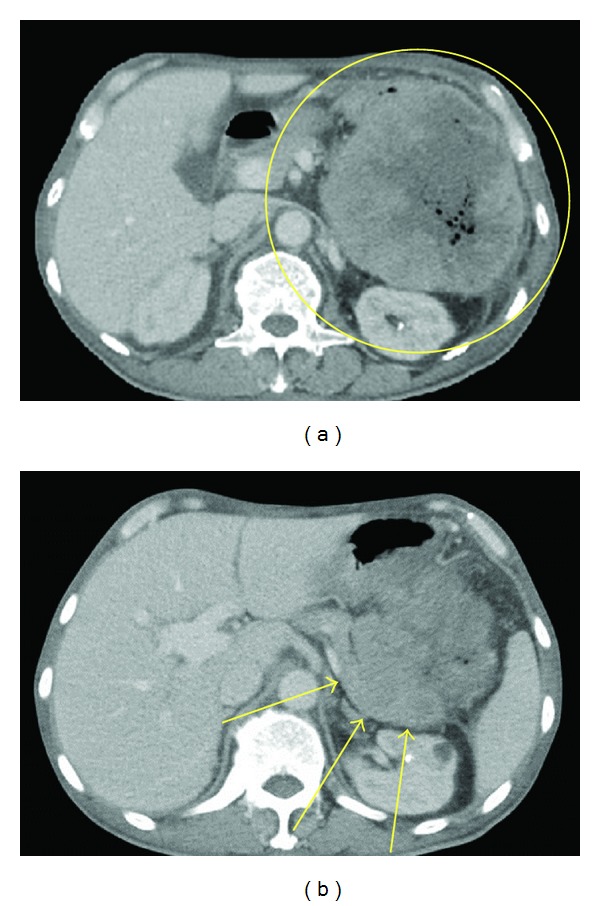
Preoperative abdominal computed tomography. (a) A large tumor (10.6 × 11.6 cm; enclosed by yellow circle) at the splenic flexure with an irregularly thickened wall and involving the tail of the pancreas; (b) tail of the pancreas (arrows).
